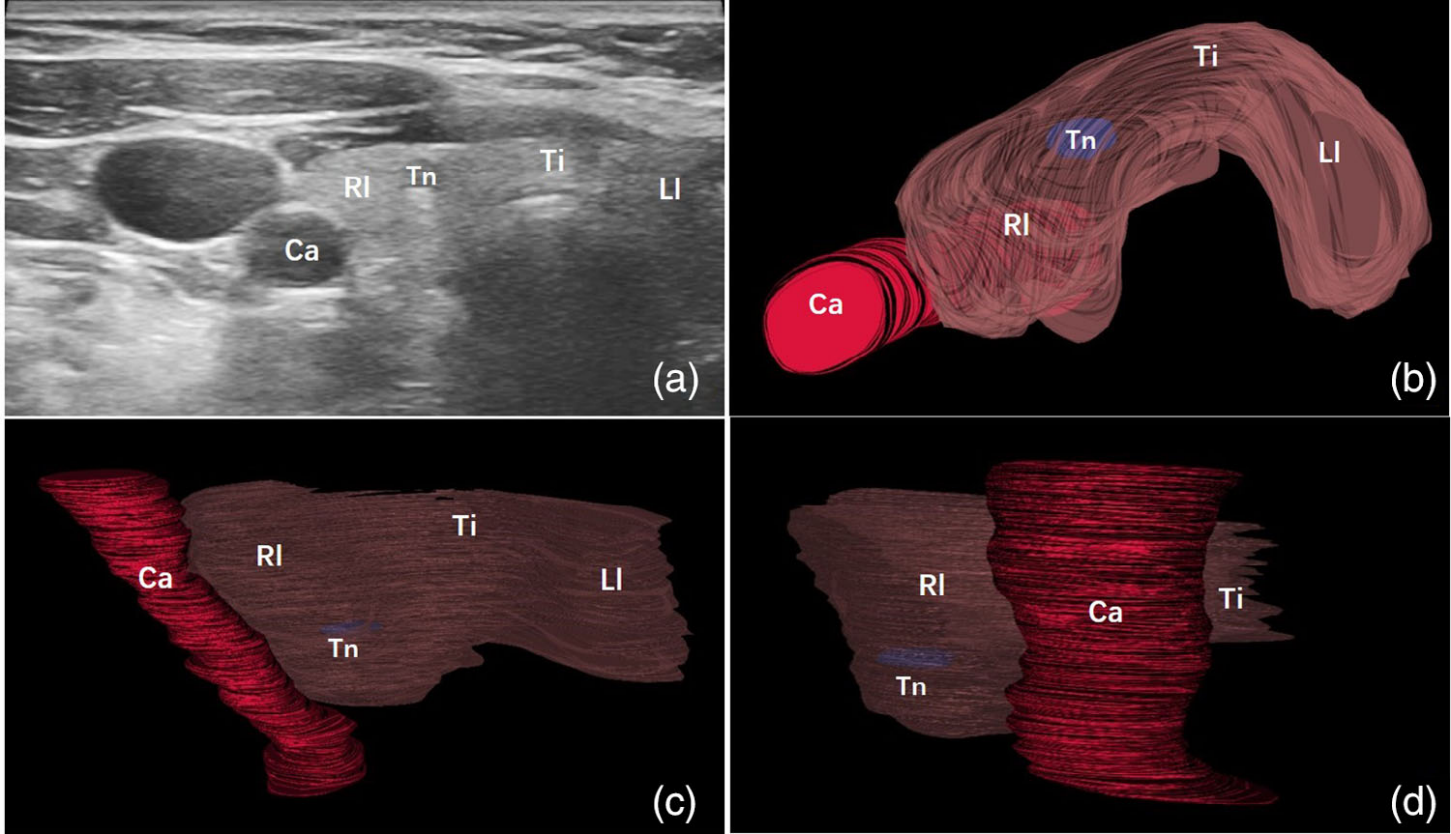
Images of a 26‐year‐old man with a thyroid nodule as small as 3 mm. The grayscale ultrasound image showed a thyroid nodule in the right thyroid lobe (a). The top view (b), front view (c), and side view (d) images from the 3D‐reconstructed model were available. Ca, carotid artery; Rl, right lobe of thyroid gland; Ti, thyroid isthmus; Tn, thyroid nodule.
